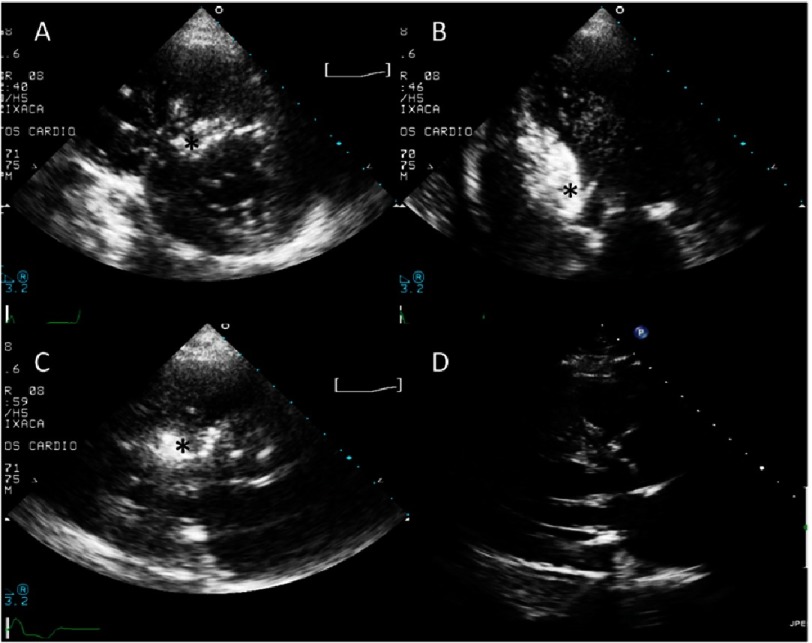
Contrast echocardiography. Multiple projections: A. Paraesternal short axis. B. Apical four-chamber. C. Paraesternal long axis. * Opacification of the targeted area of the septum. D. Paraesternal long axis after septal ablation.
